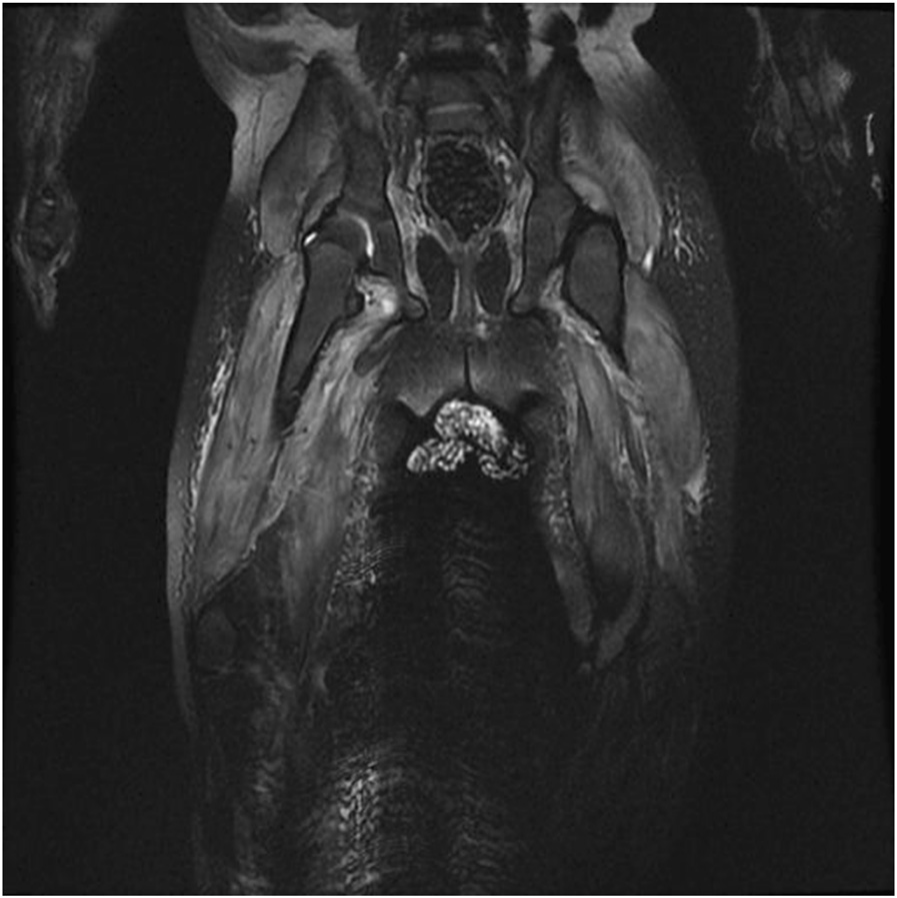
Coronal T2 magnetic resonance imaging sequence of upper legs, showing muscle and subcutaneous oedema.
